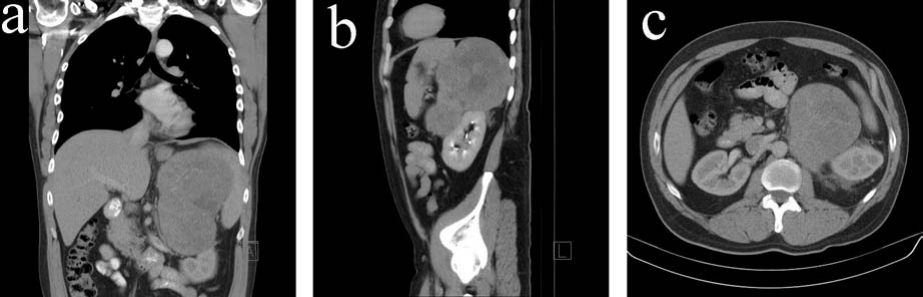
(a) CT scan (coronal view) on initial diagnosis. (b) CT scan (sagittal view) on initial diagnosis. (c) CT scan (transverse view) on initial diagnosis.
